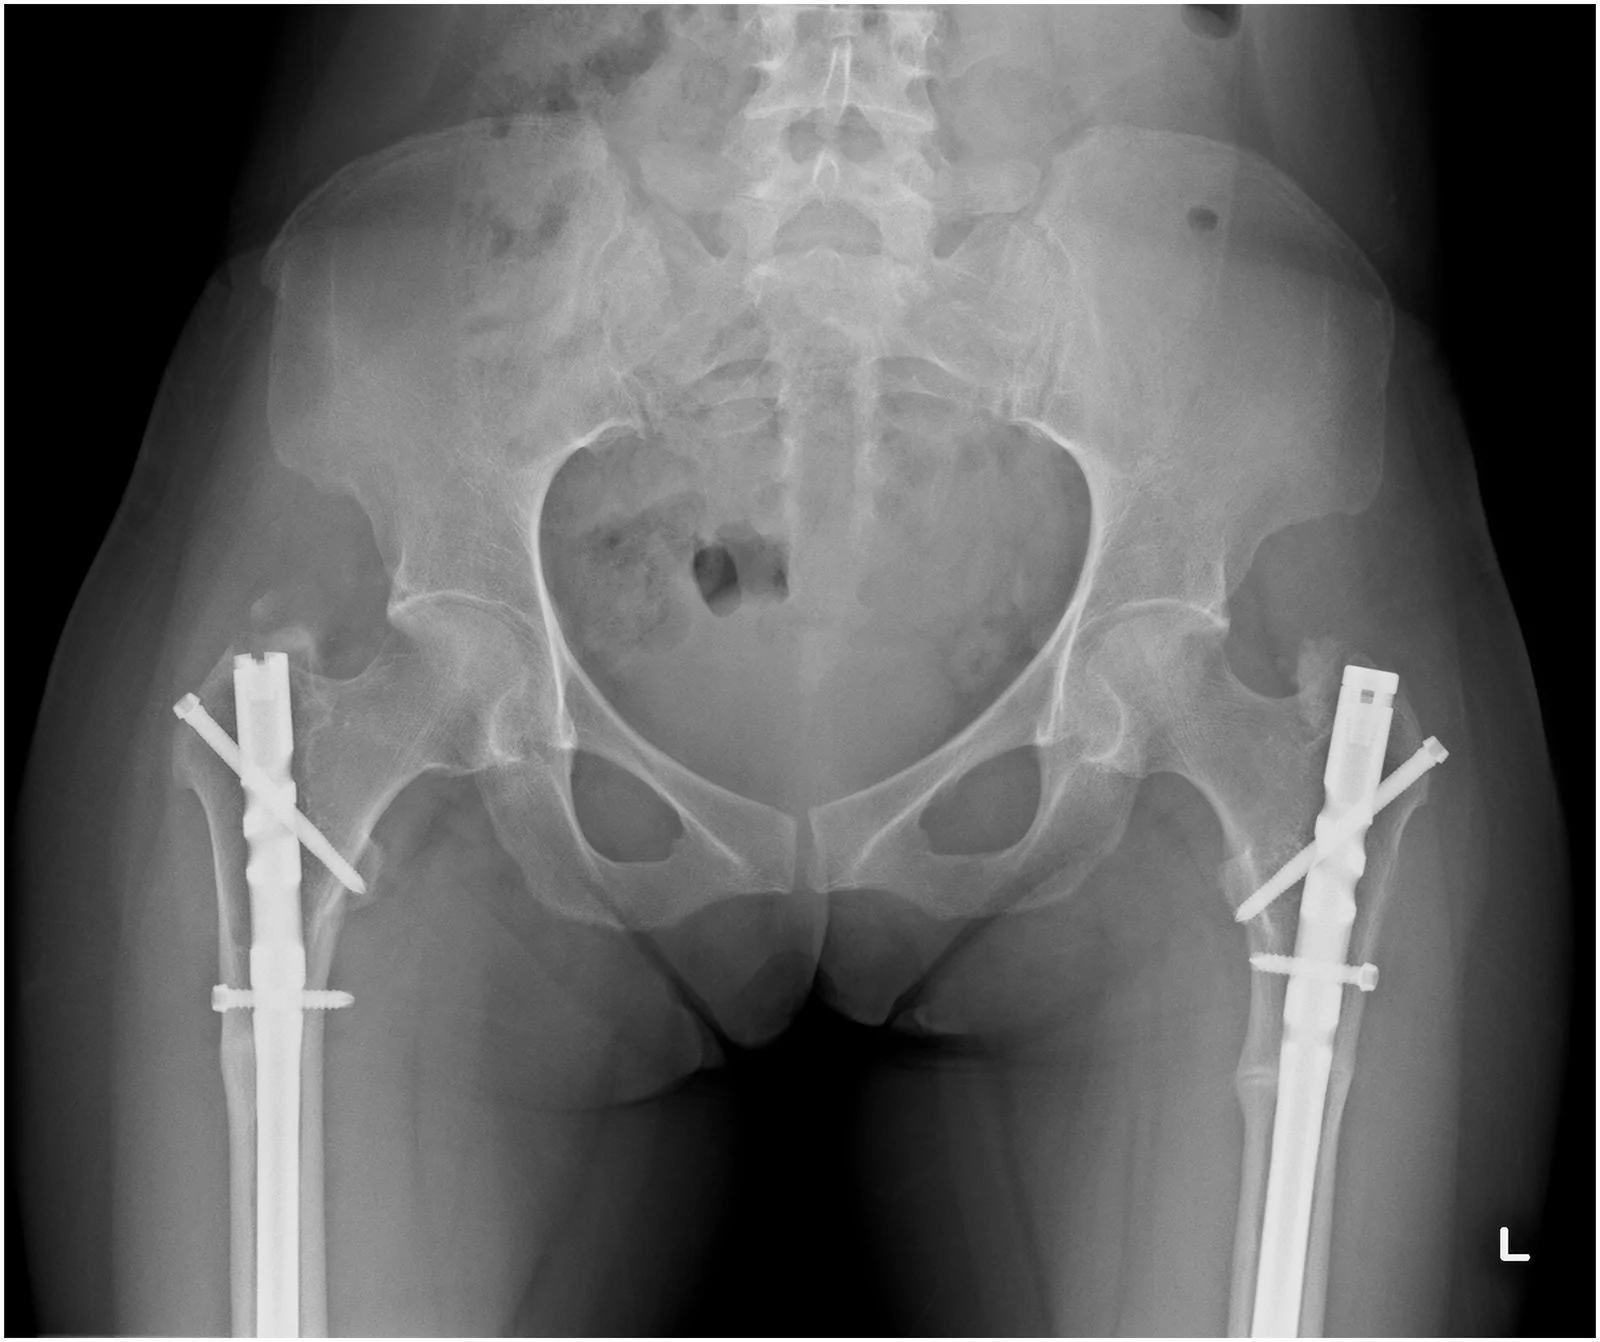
Radiographic finding of the mother. Anteroposterior radiograph of the mother's hips at age 32, with intramedullary nails inserted for femoral fractures, demonstrating the severity of her bone disease prior to burosumab treatment.
